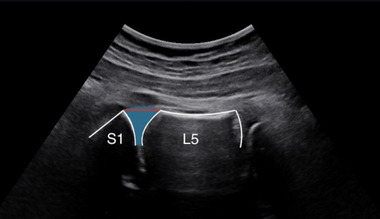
Long‐axis ultrasonic scanning L5–S1 anterior intervertebral disc height.
